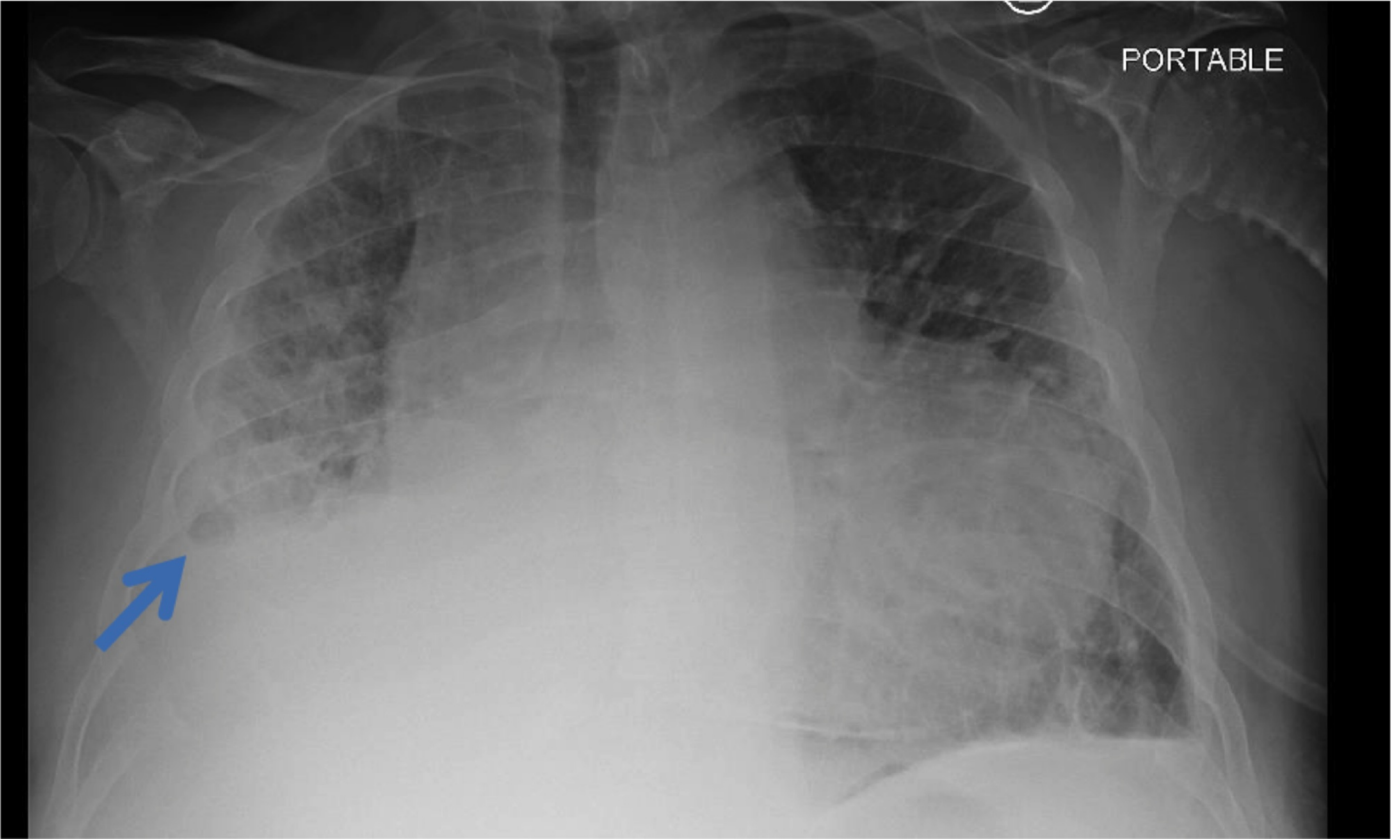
Chest X-ray (CXR) showing collapse of right middle and lower lobes
